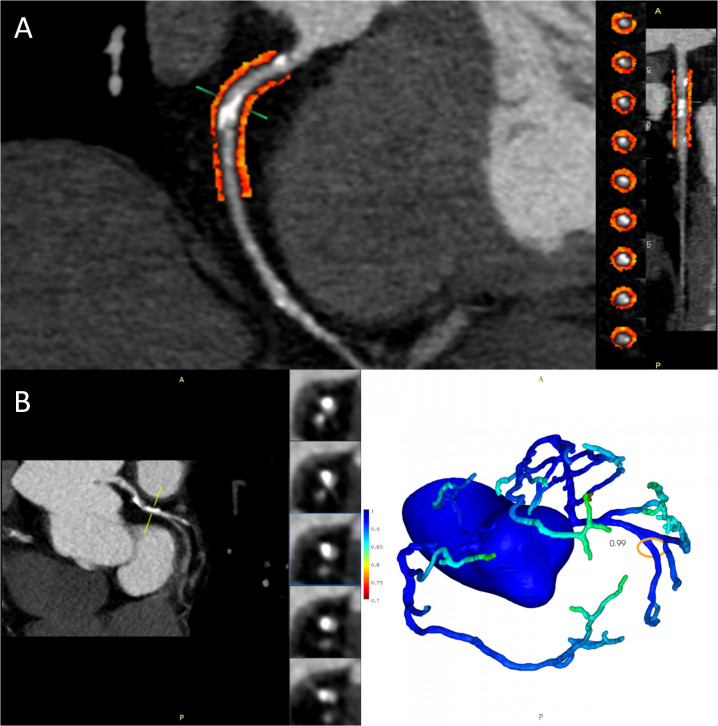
Imaging techniques used for the measurement of key cardiovascular parameters in MAFLD and CHD patients. (A) The computed tomography angiography surface plane reconstruction image, combined with fat segmentation, is used for the measurement of Fat Attenuation Index. (B) The computed tomography angiography surface plane reconstruction image and VR coronary artery tree color map are used for the measurement of CT fractional flow reserve values.
